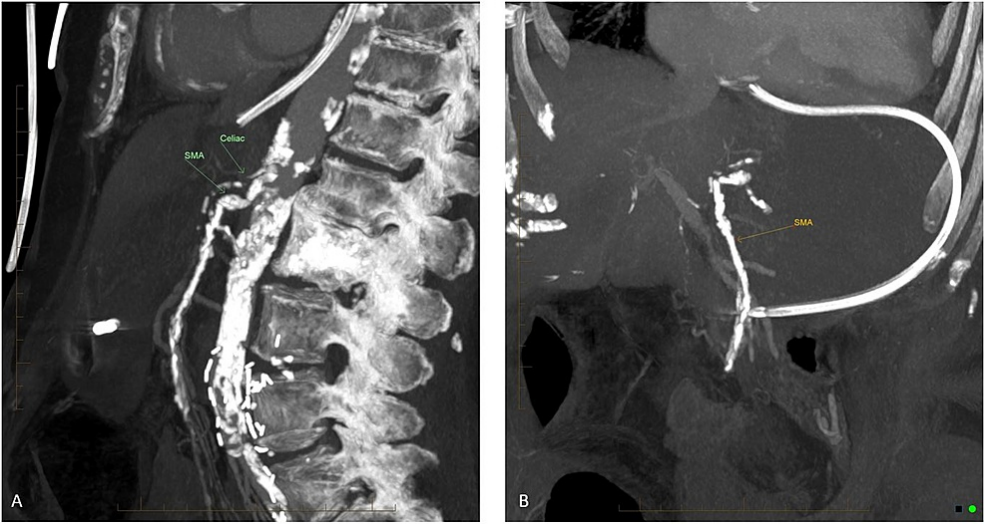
Sagittal view (A) and (B) CT abdomen and pelvis demonstrating occlusion of SMA and celiac artery with the absence of contrast. SMA, superior mesenteric artery
